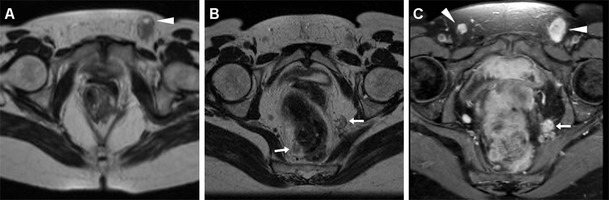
The same patient as in Fig. 2. Axial T2-weighted (a,b) and post-contrast fat-suppressed T1-weighted (c) images show inguinal nodal metastases, larger on left side (*arrowheads*) plus bilateral enhancing perirectal adenopathies (*arrows*)
